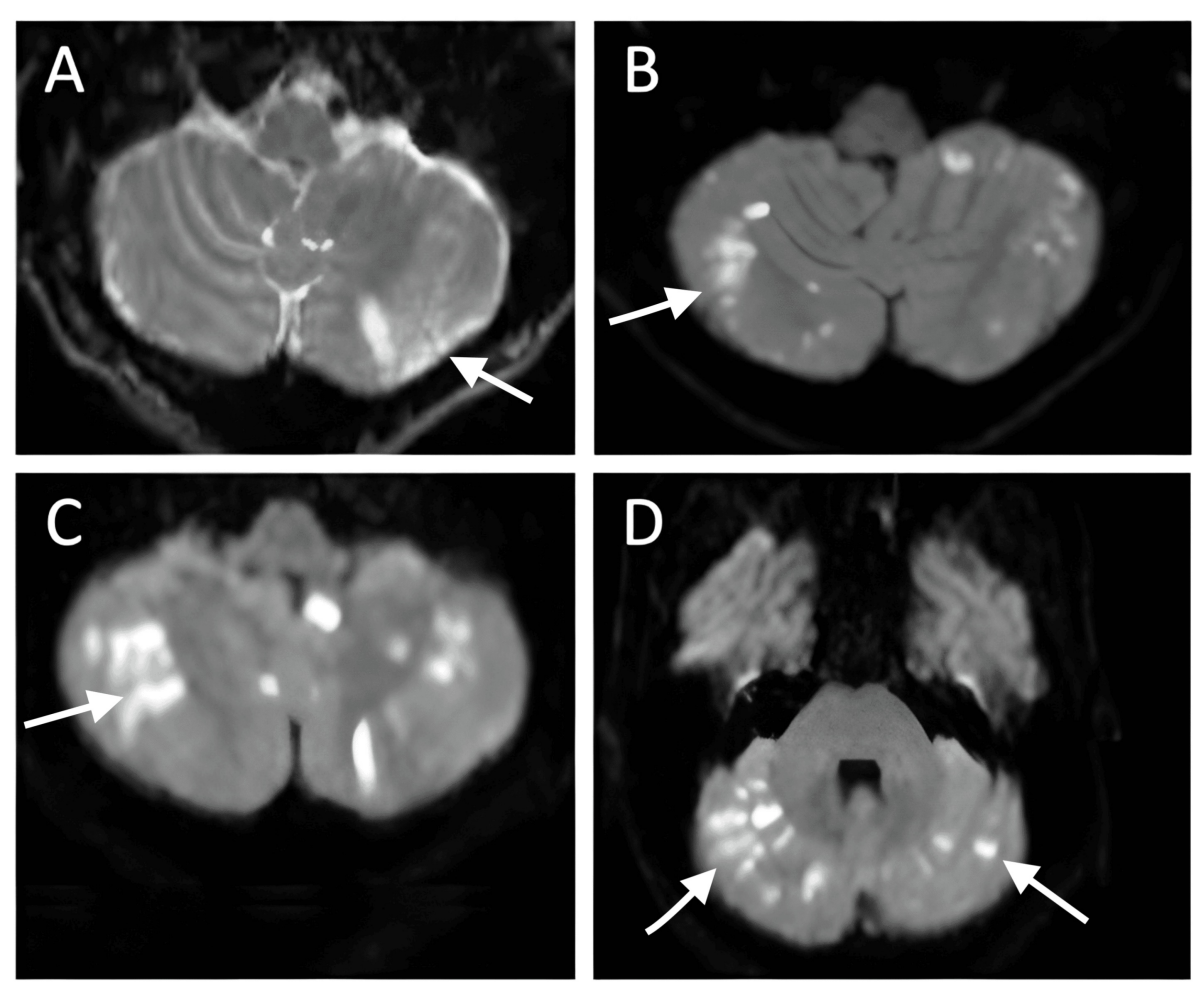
Axial diffusion weighted MRI scans in a 70-year-old male patient following the diagnosis of cryptococcal meningitis from initial presentation showing infarcts in the posterolateral cerebellar hemisphere (A). More numerous diffusion positive infarcts involving both cerebellar hemispheres are seen at 2 weeks (B), 3 weeks (C,D)
